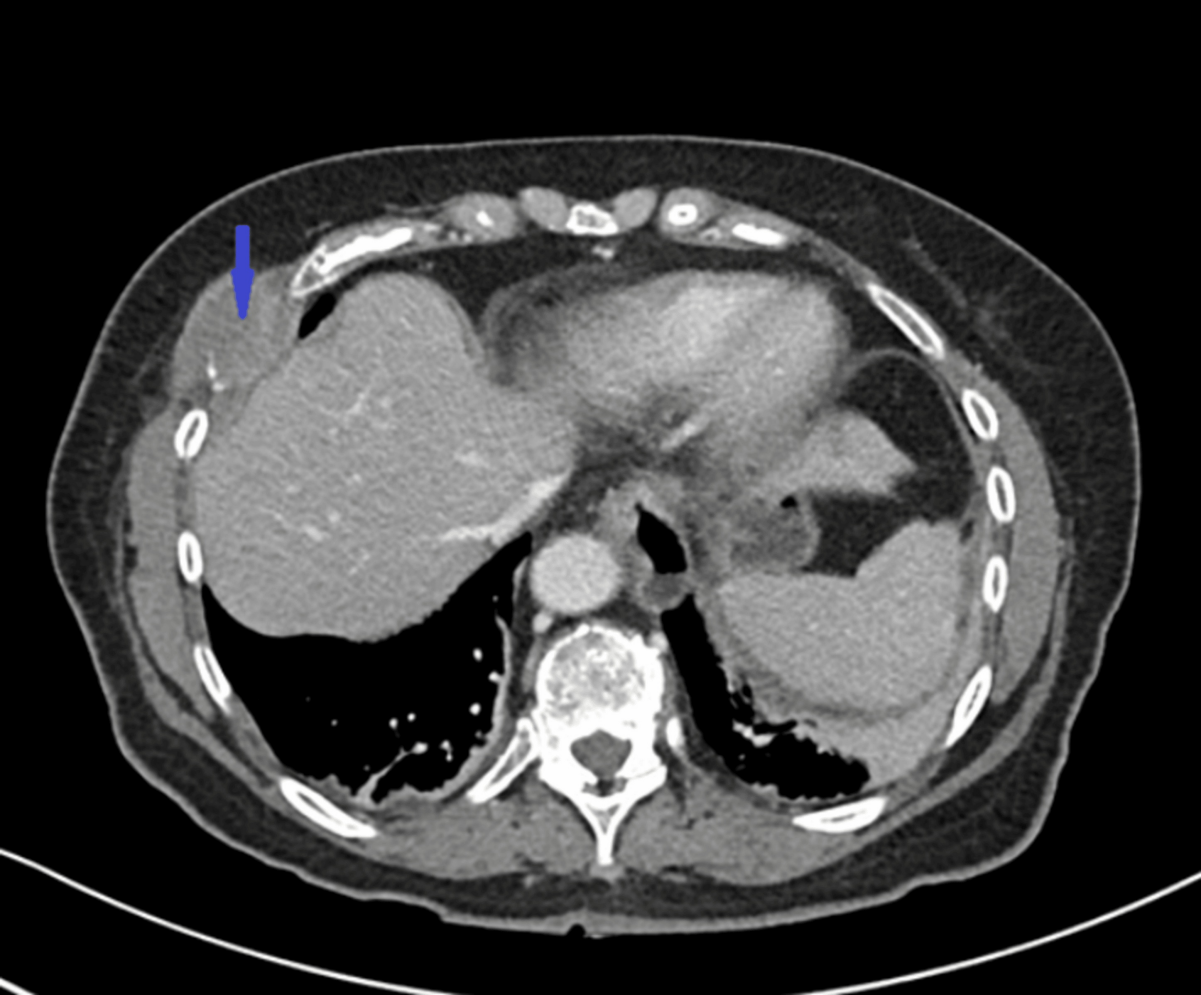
Cross-sectional CT image of the chest showing lytic lesion in the right sixth vertebrae (blue arrow)
